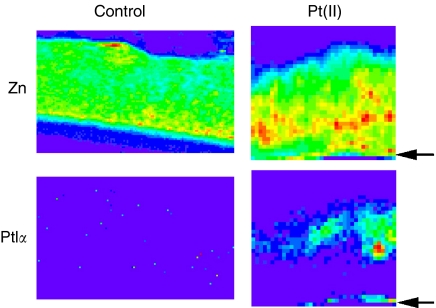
The cellular accumulation of [^14^C]-Pt(IV) in MCL: SRIXE analysis. After the transport assay the MCL was processed, embedded in wax and 20 *μ*m sections through the MCL were imaged in a synchrotron x-ray beam using SRIXE. Fitted images of the elemental distributions in segments of a Pt(IV)-treated MCL and a control non-treated MCL are shown. The scale on the axes represents the number of pixels, where each pixel is 3 × 2 *μ*m (horizontal x vertical). The images show relative elemental concentrations, using the colour scale shown, which ranges from blue, representing low levels, to red, representing high elemental levels. The arrowheads indicate the position of the PTFE membrane supporting the MCL (shown in Figure 1D).
